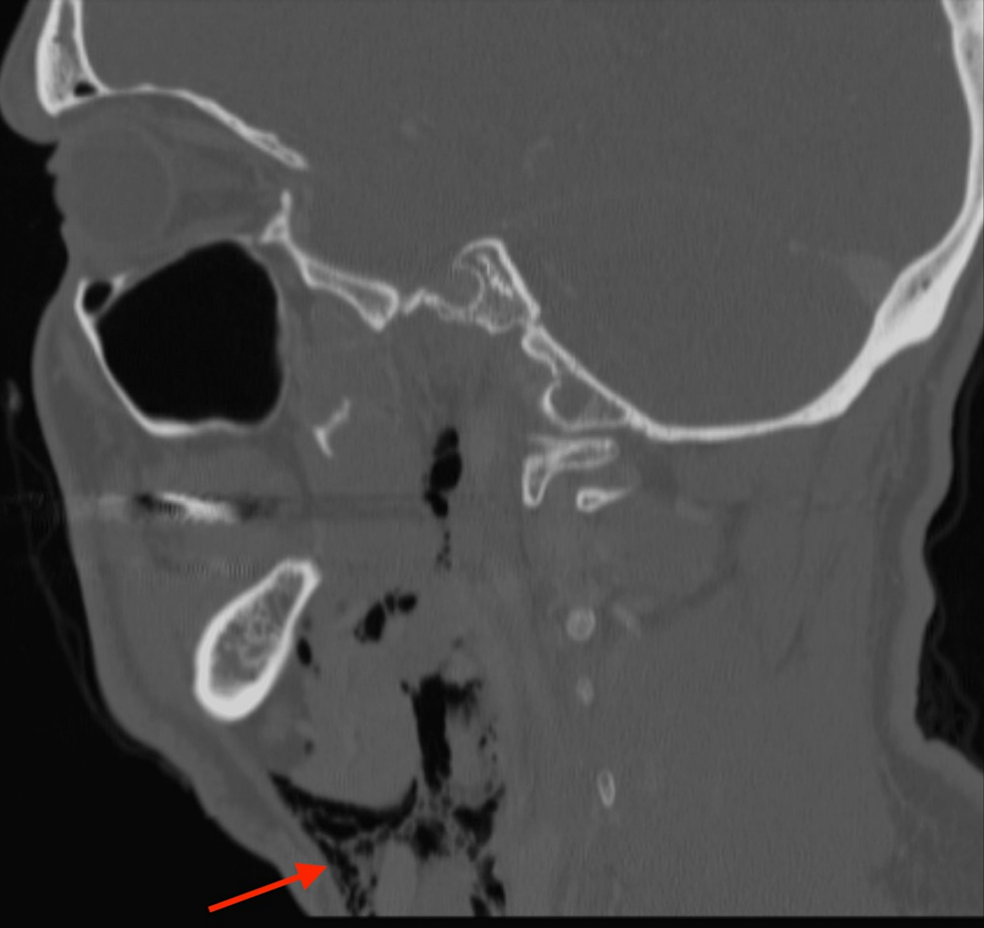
CT Maxillofacial, sagittal view Red arrow: subcutaneous emphysema extending inferiorly through the soft tissues of the neck
